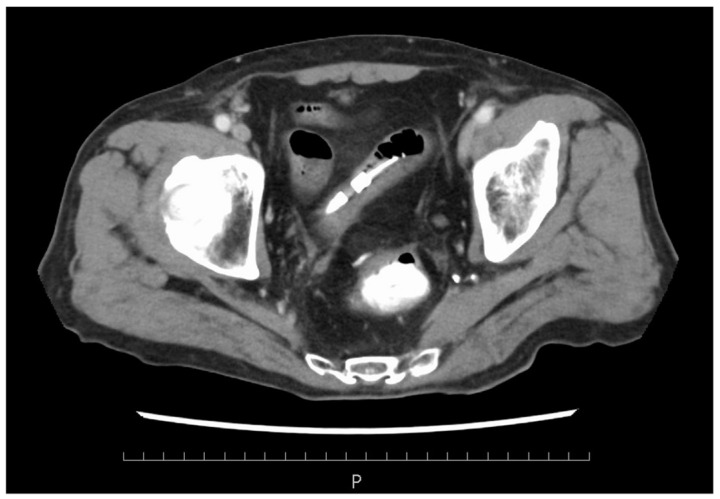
Abdominal contrast-enhanced CT showed no obvious anastomotic leakage, and the bowel obstruction had improved.
